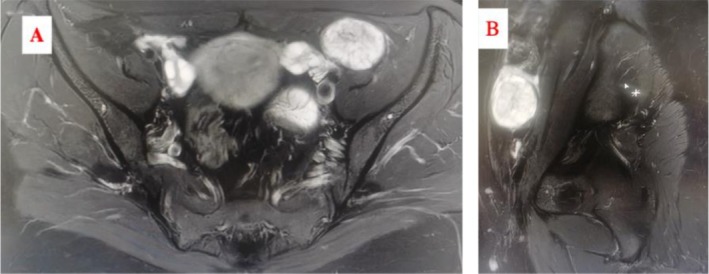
MRI demonstrated an inhomogeneous ectopic mass in both the (A) axial and (B) coronal section.
